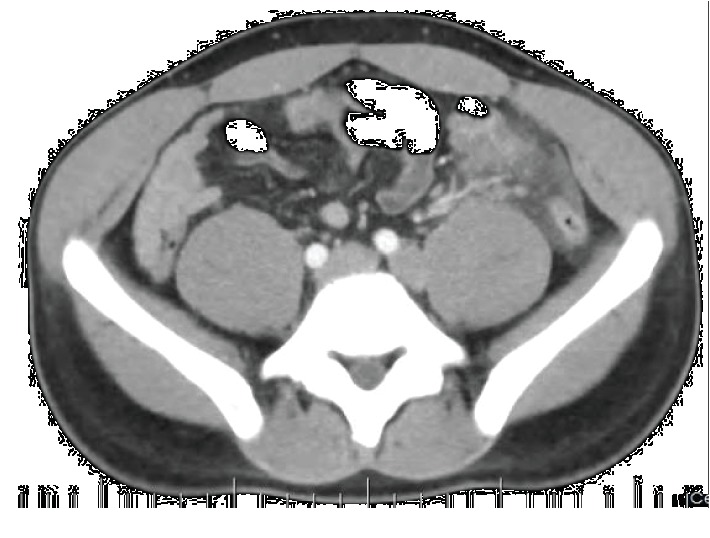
Computed tomography scan axial cut evidencing blurring view of the adjacent visceral fat in the left iliac fossa.
